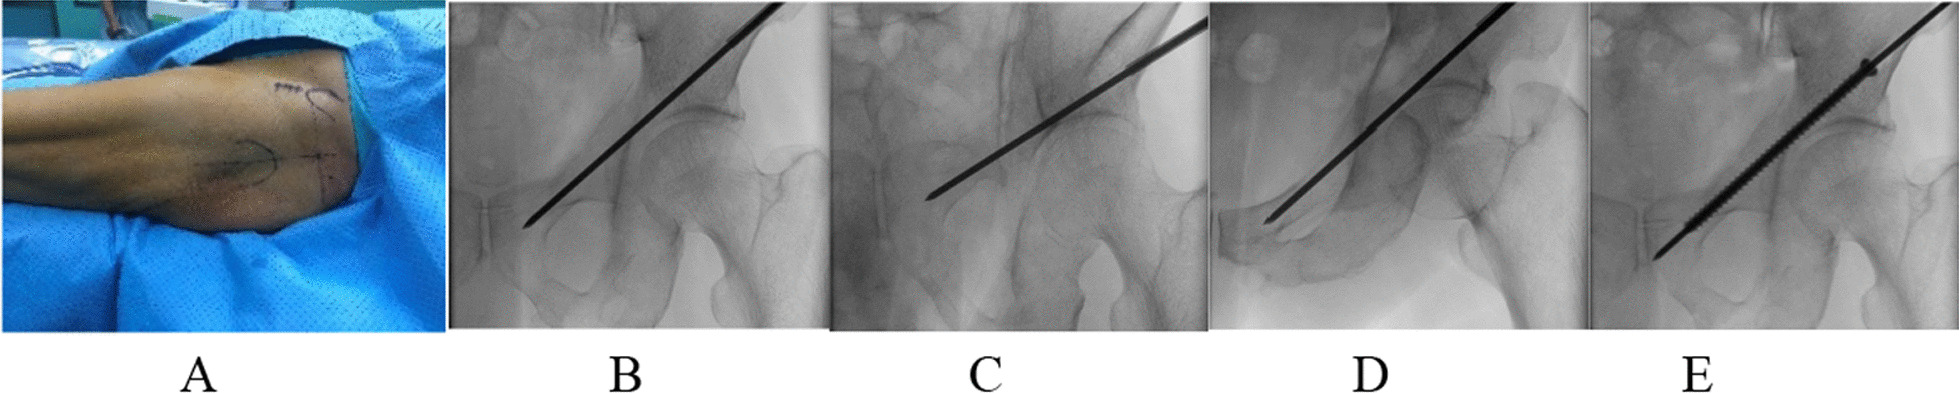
Insert the anterior column screw (A take the upper extension of the apex of the greater trochanteric of the femur and the horizontal intersection of the anterior superior iliac spine at the needle entry point and insert the needle in the direction of the pubic symphysis; B–D pelvic orthography, exit radiograph, and entrance position determine that the guide needle does not penetrate the cortex; E Insert the screw along the guide pin)
